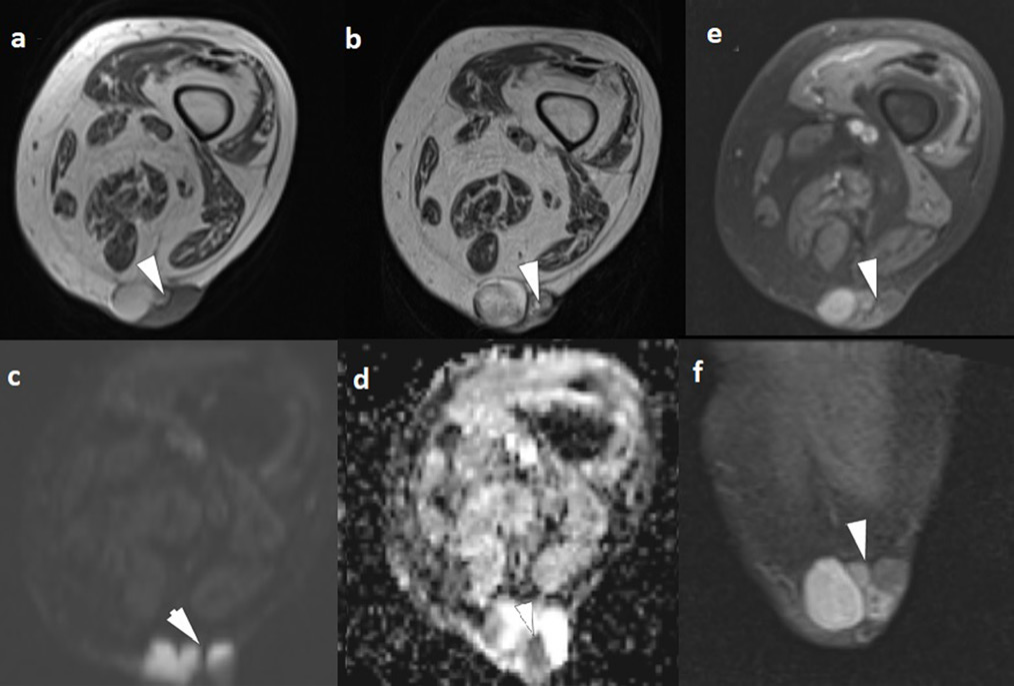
MRI of the mass in axial *T*
